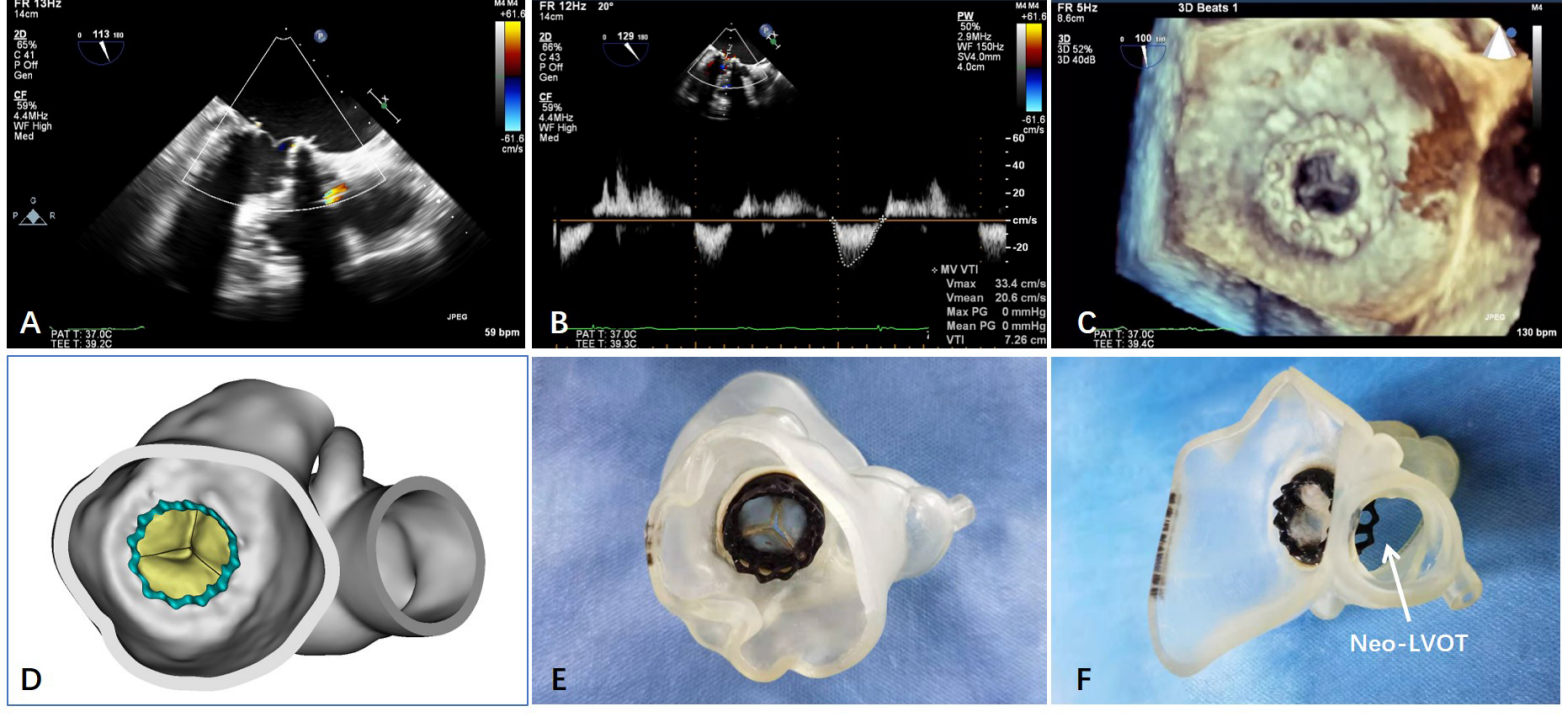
Postoperative transthoracic echocardiograms, CT scans and 3D
